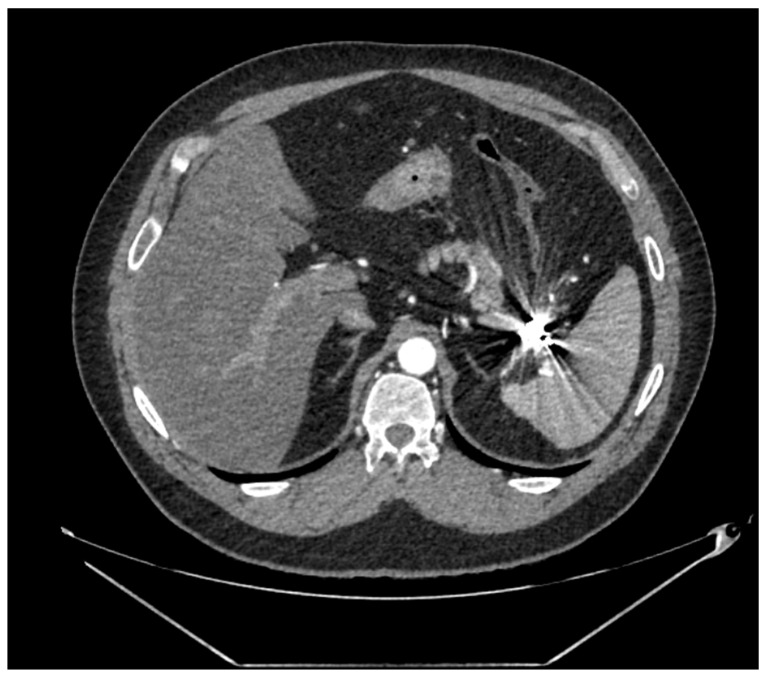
Post-operative CTA following coil embolization of a splenic artery aneurysm.
